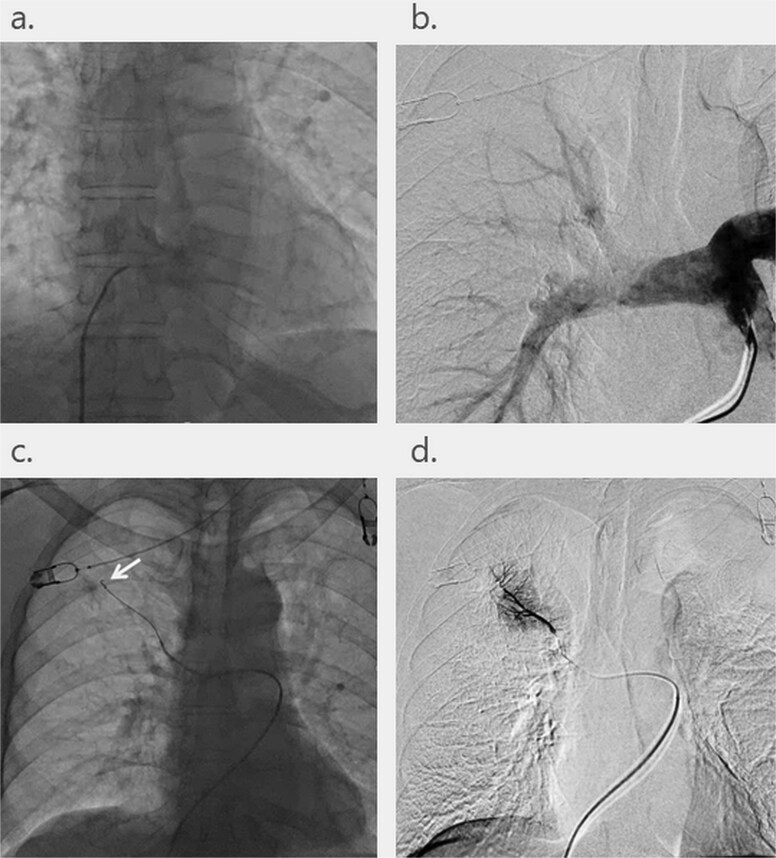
Technique of transcatheter pulmonary arterial fiducial marker placement. (a) A guiding catheter is inserted into the inferior vena cava via the right femoral vein with ultrasound echocardiography guidance. (b) A 6Fr headhunter-type catheter is placed into the pulmonary artery via the right atrium and ventricle through a guiding catheter. (c) An embolic platinum coil is placed as a fiducial marker into a peripheral pulmonary artery near the tumor by using a micro-catheter through a guiding catheter. (d) Digital subtraction angiography from the micro-catheter.
